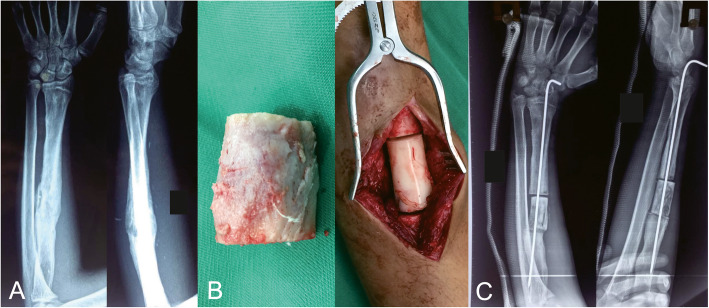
A 25-year-old man who suffered osteomyelitis in his left radius and treated by bone transport technique. a Preoperative AP and lateral views of X-rays. b Debridement of the infected site with a 4 cm bone defect in left radius and antibiotic bone cement was used to occupy the residual space. c Postoperative AP and lateral views of x-rays, the forearm was fixated by Kirschner wire and plaster cast
